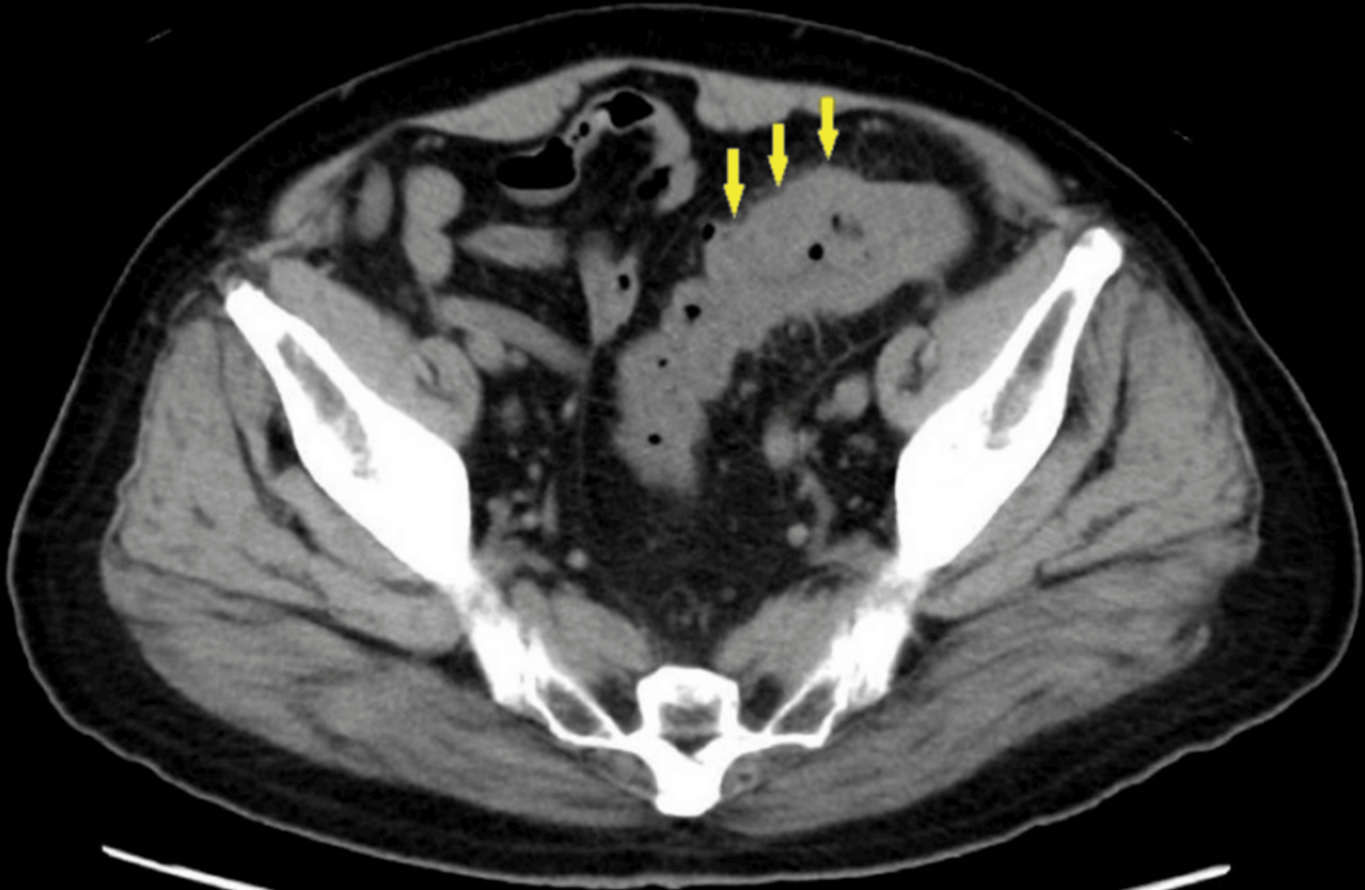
Plain abdominal CT scan Wall thickening of the sigmoid colon was apparent (yellow arrow).
